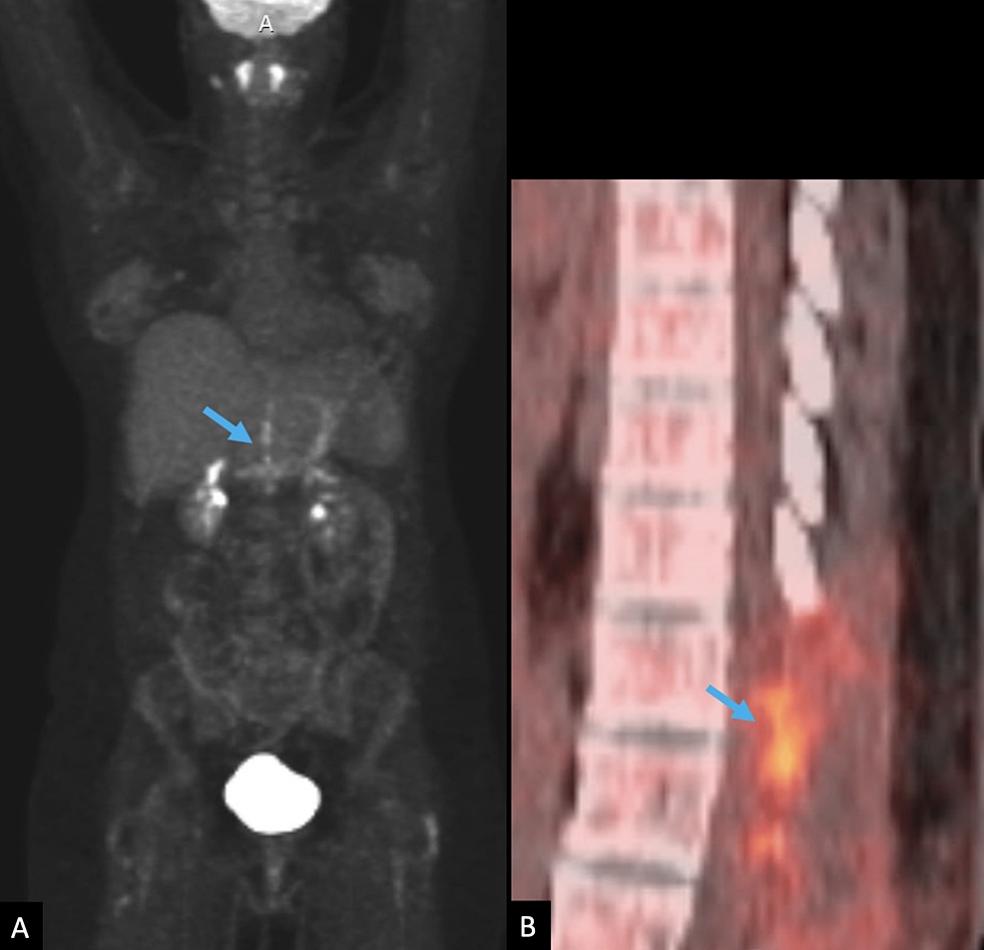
Postoperative PET/CT imaging A PET/CT, approximately two months following the initial preoperative MRI in 2020. Whole-body maximum intensity projection (MIP) PET (A) and sagittal (B) fused PET/CT show linearly increased F-fluorodeoxyglucose (FDG) uptake at the surgical site, representing residual tumor and postoperative change (blue arrows). There is no malignant FDG-avid disease elsewhere on the exam.
